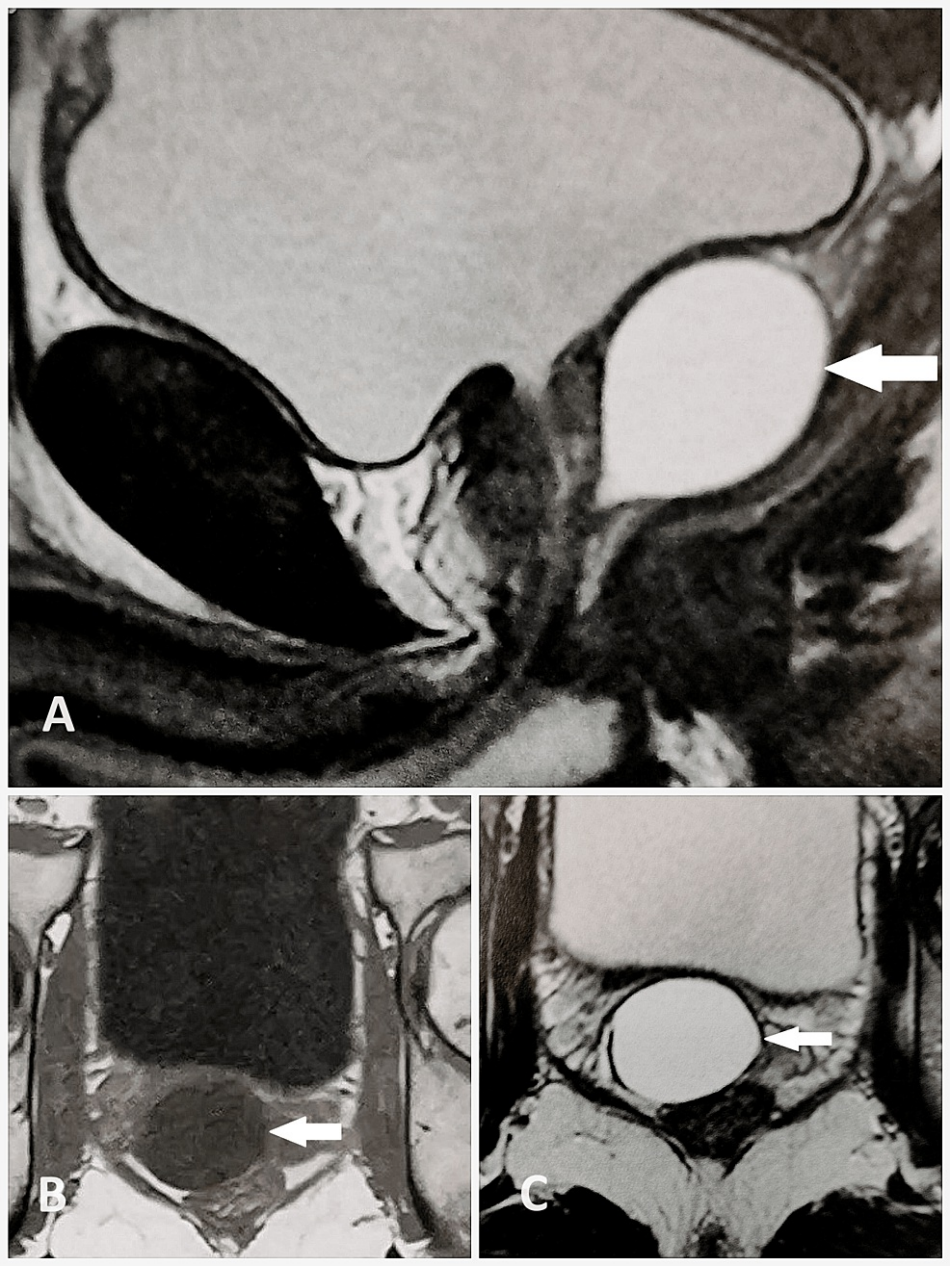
MRI image showing a round cystic mass in the prostate. A: Sagittal view of the T2-weighted sequence. B: Axial view of the T1-weighted sequence. C: Axial view of the T2-weighted sequence.
